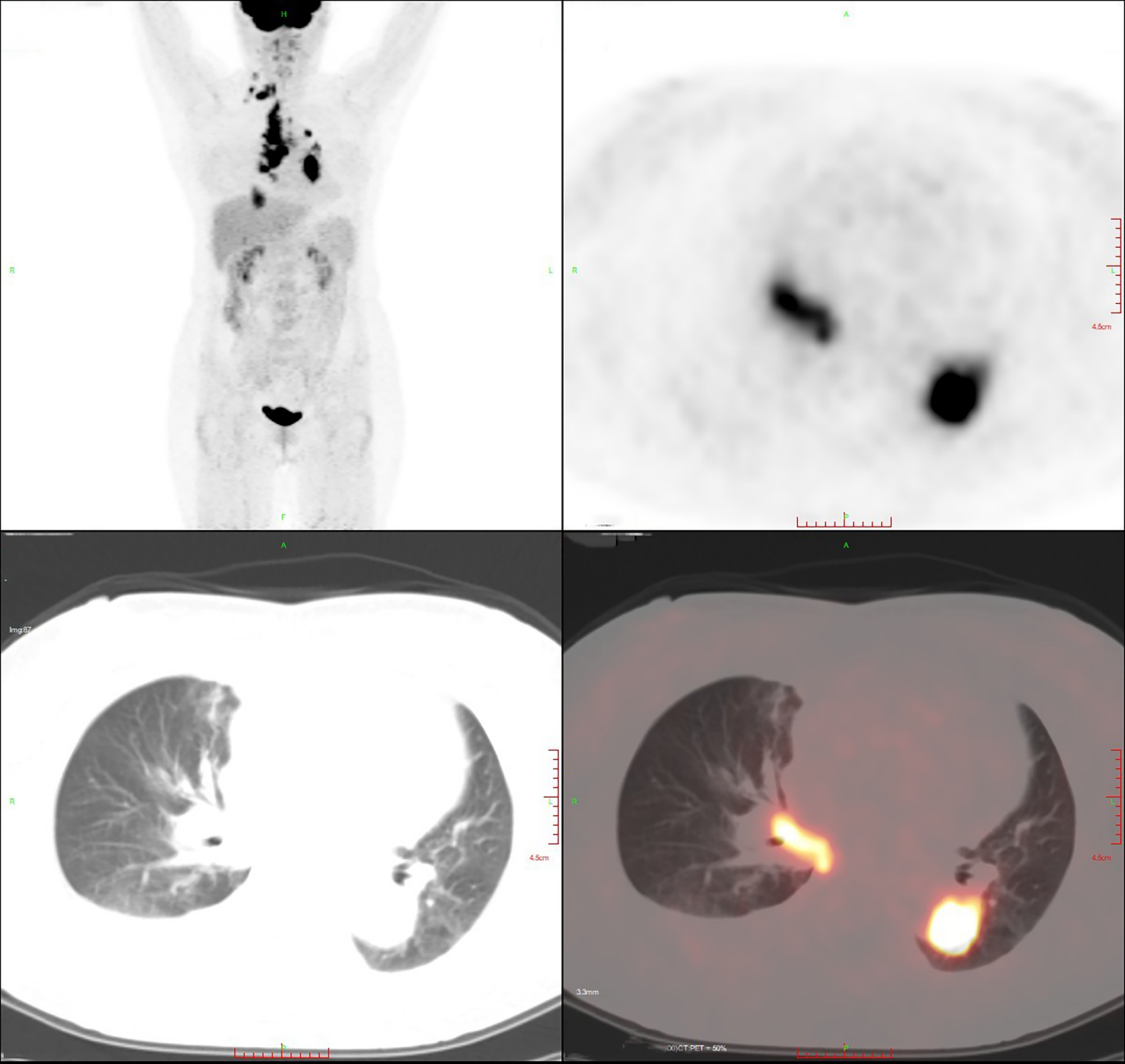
The lesions on positron emission tomography/computed tomography (PET/CT).
